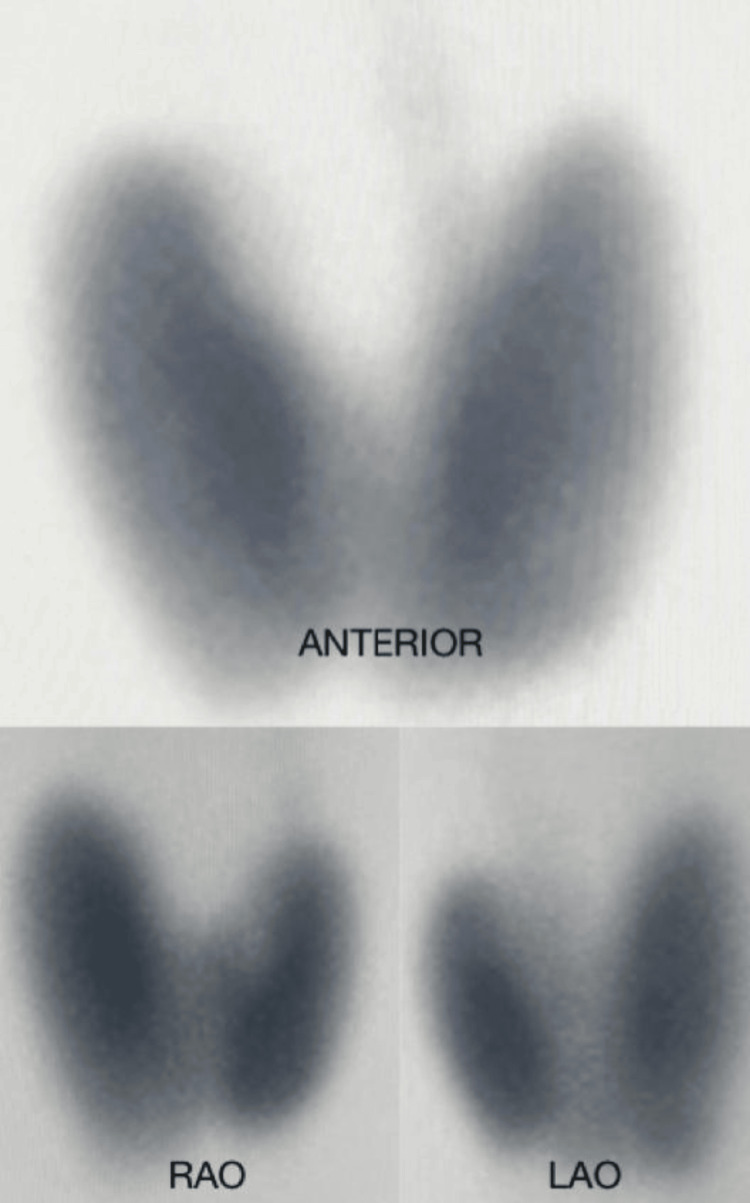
Thyroid scintigraphy (technetium-99m sodium pertechnetate) showing an enlarged thyroid gland with homogeneous, globally increased uptake, with no focal hot or cold nodules.
